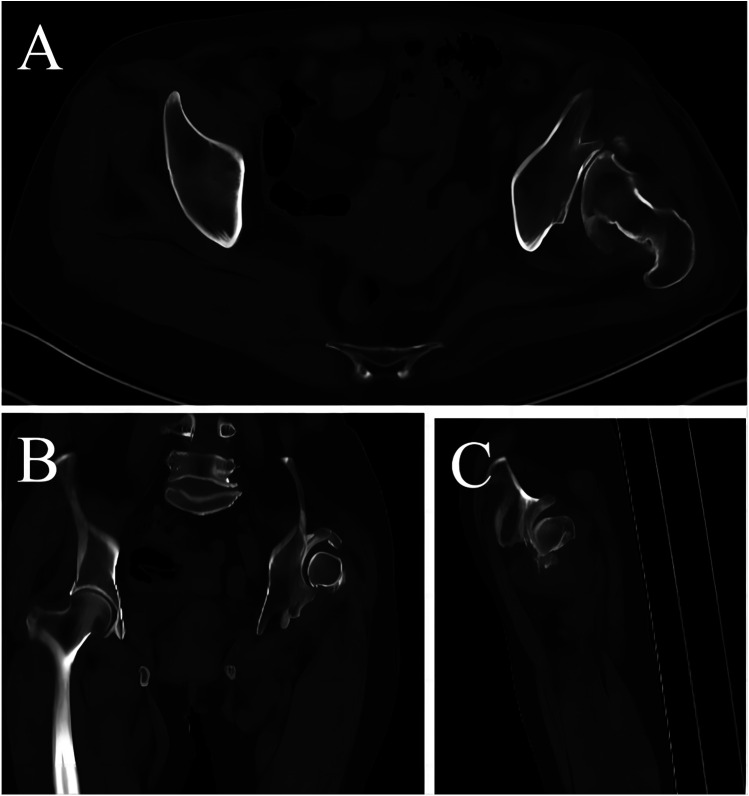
Different types of CT reformation techniques for long-term unreduced posterior hip dislocation. (A) Axial CT. (B) Coronal multiplanar reformation. (C) Sagittal multiplanar reformation.
